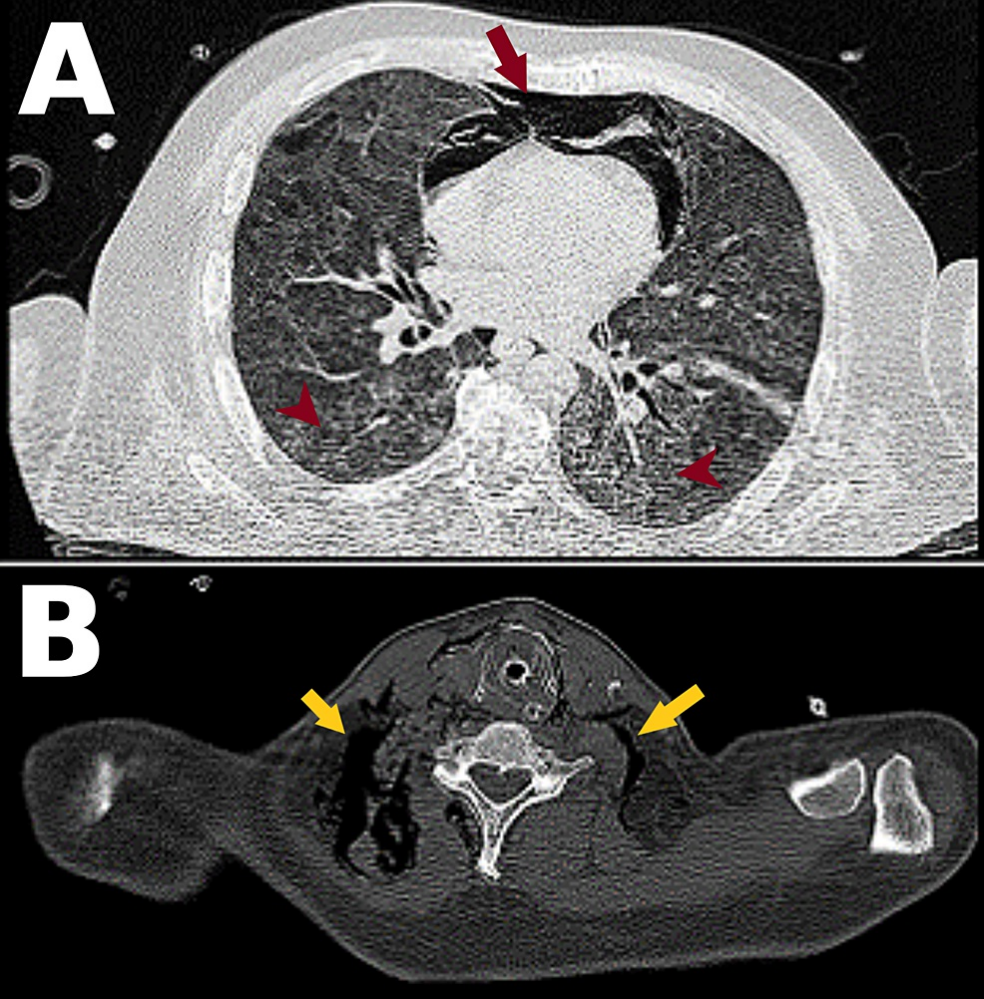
Non-enhanced CT scan of the chest. A: Lung view showing diffuse bilateral ground-glass opacities (red arrowheads) and pneumomediastinum (red arrows). B: Mediastinal view showing subcutaneous emphysema (yellow arrows).
